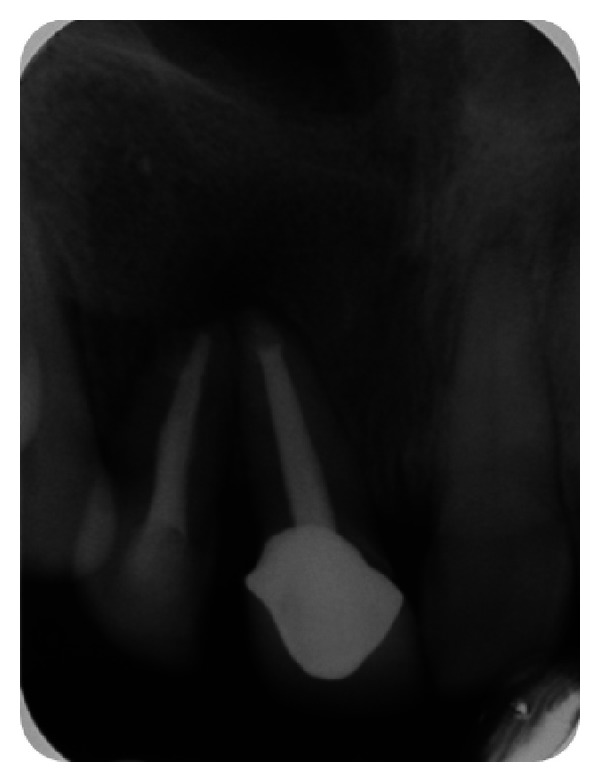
24-month followup IOPA radiograph.
